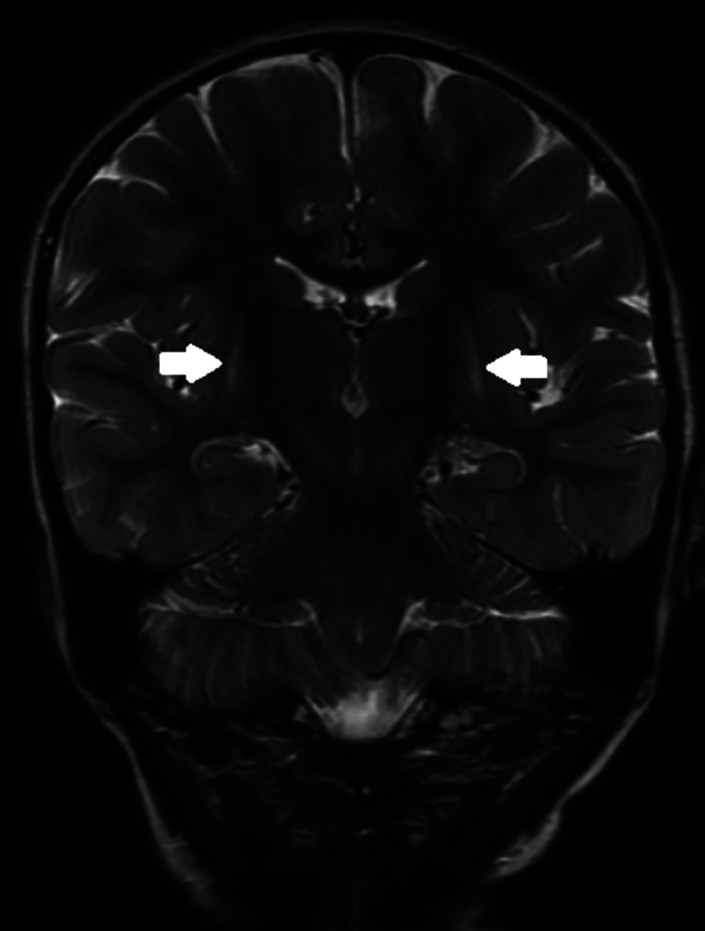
MRI T2 imaging showing subtle posterior putamen hyperintensity (white arrows)
